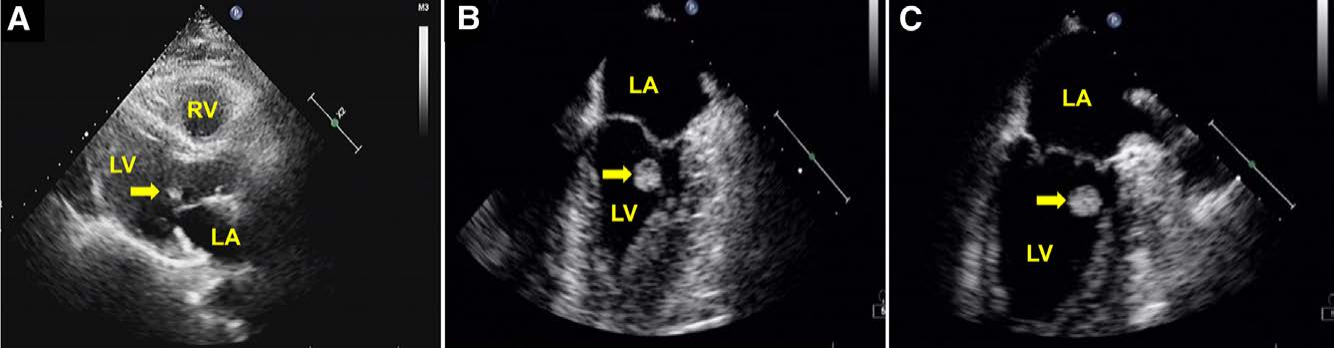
(A) Two-dimensional transthoracic echocardiography showing a floating round-shaped hyperechoic mass on the anterior mitral valve (yellow arrow). (B, C) Transesophageal echocardiography showing a regular, round tumor (yellow arrow) that appeared to be attached to the anterior mitral valve leaflet by a small pedicle. LA = left atrium, LV = left ventricle, RA = right atrium.
